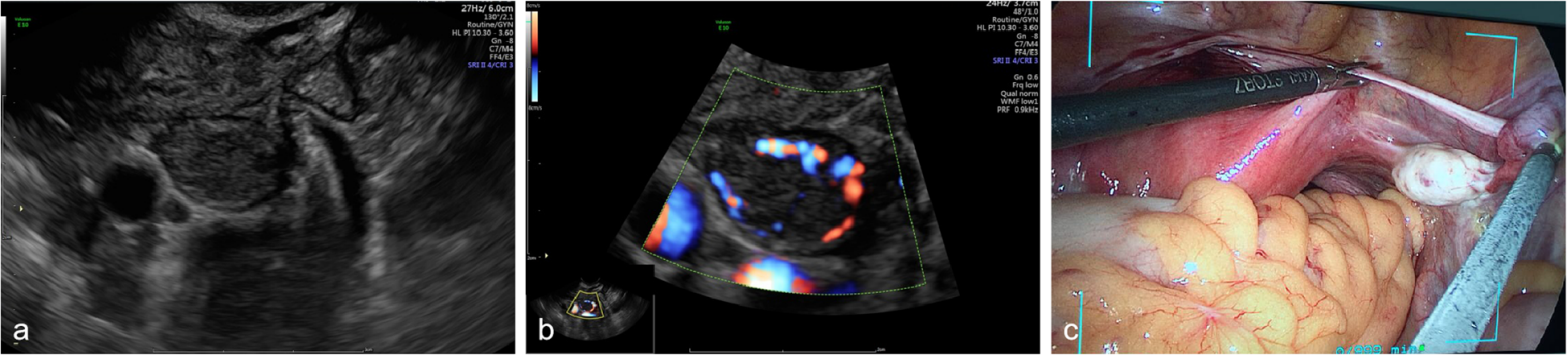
A 69-year-old woman suffered from LCT. a A solid mass with clear border was detected in the right ovary by ultrasound examination; b CDFI findings showed the mass with abundant doppler flow signals; c Intraoperativel findings showed right ovarian was hard, the surface smooth, having a good mobility
